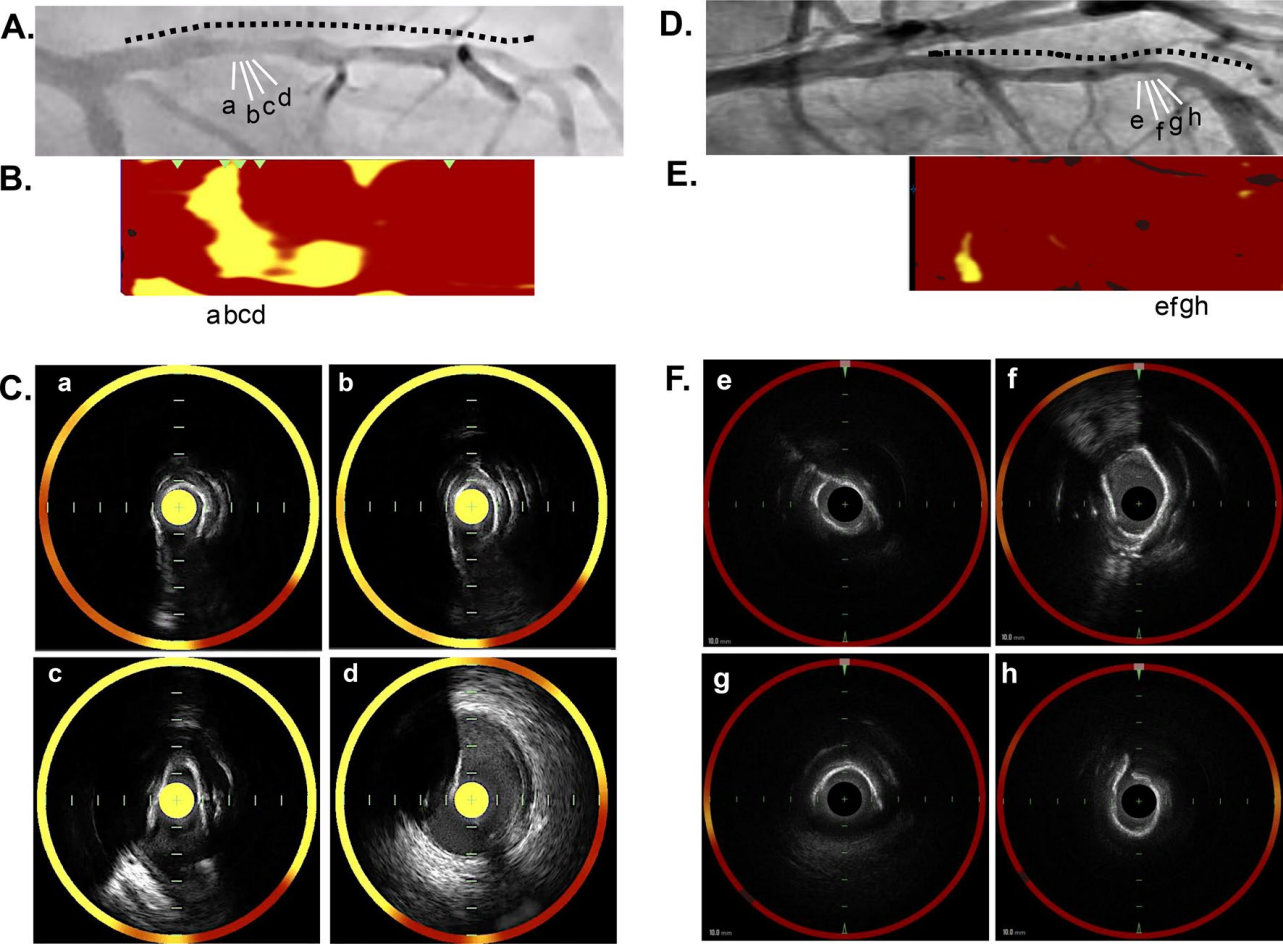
Representative cases. A A 67-year old gentleman presented stable angina pectoris. The proximal segment of his LAD was treated by PCI. Black dotted line was the imaged segments with NIRS/IVUS. a, b, c and d correspond to images in B and C. B NIRS imaging prior to PCI visualized the presence of lipidic plaque materials at the calcified target lesion, and maxLCBI_4mm_ was 817. C Cross-sectional images (D) showed the extensive yellow signals at the corresponding calcified lesion. The maximum calcification arc was 297°. After stent implantation, no-reflow phenomenon occurred. D A 58-yearl old gentleman received an elective PCI to treat moderate stenosis at the middle segment of his LAD. Black dotted line was the imaged segments with NIRS/IVUS. e, f, g and h correspond to images in E and F. E MaxLCBI_4mm_ at this lesion was 83. F On NIRS/IVUS cross-sectional imaging, calcification arc was 360° (H), accompanied by very small amount of yellow signals. No-reflow phenomenon did not occur after PCI. *IVUS* intravascular ultrasound, *LAD* left anterior descending artery, *MaxLCBI*_*4mm*_ maximum 4-mm lipid-core burden index, *NIRS* near-infrared spectroscopy, *PCI* percutaneous coronary intervention
